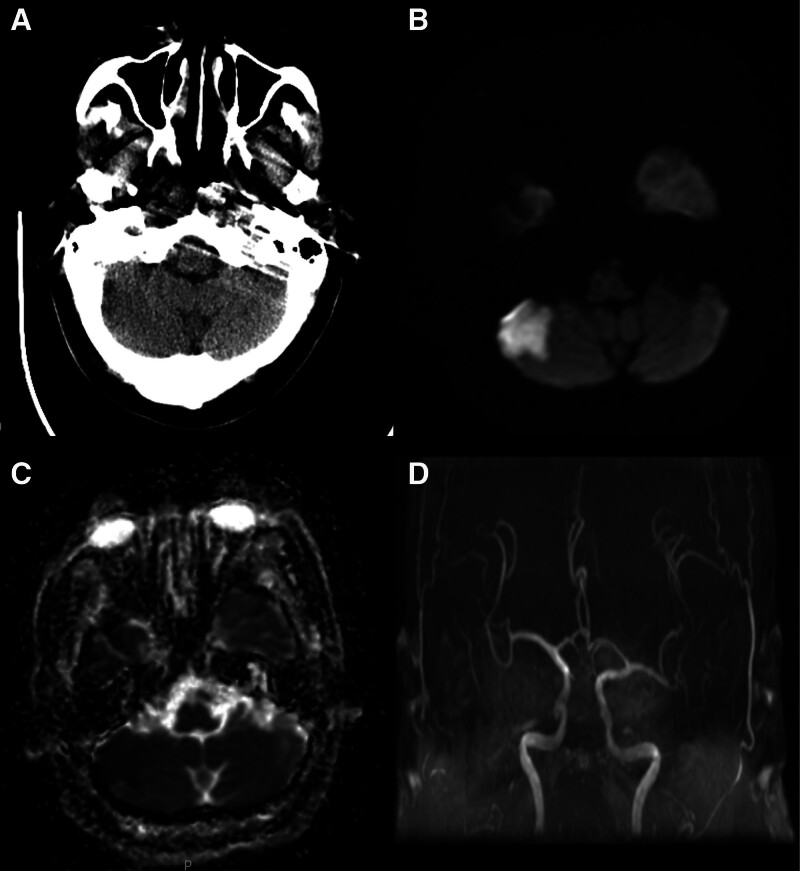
(A) Cranial computed tomography (CT) showed no evidence of intracranial hemorrhage. (B and C) Diffusion-weighted imaging and apparent diffusion coefficient images indicating acute infarcts in the right pons and cerebellar hemisphere. (D) Cranial magnetic resonance angiography (MRA) image indicating alterations suggestive of basilar artery occlusion.
